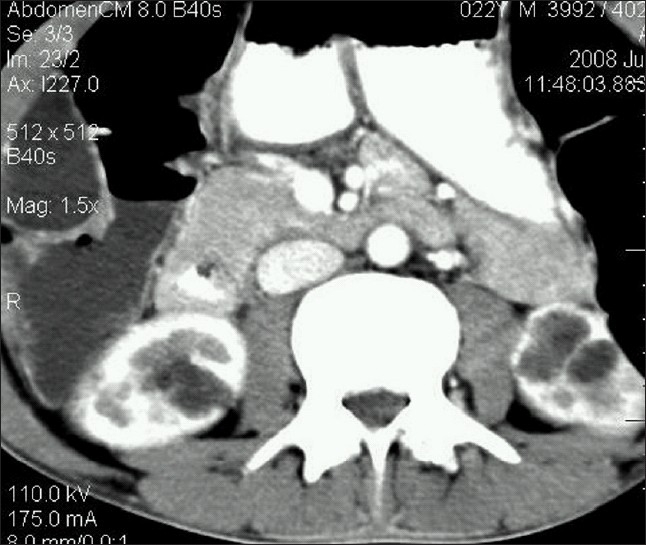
Axial contrast-enhanced computed tomography showing multiple cysts in the lower poles of both kidneys
